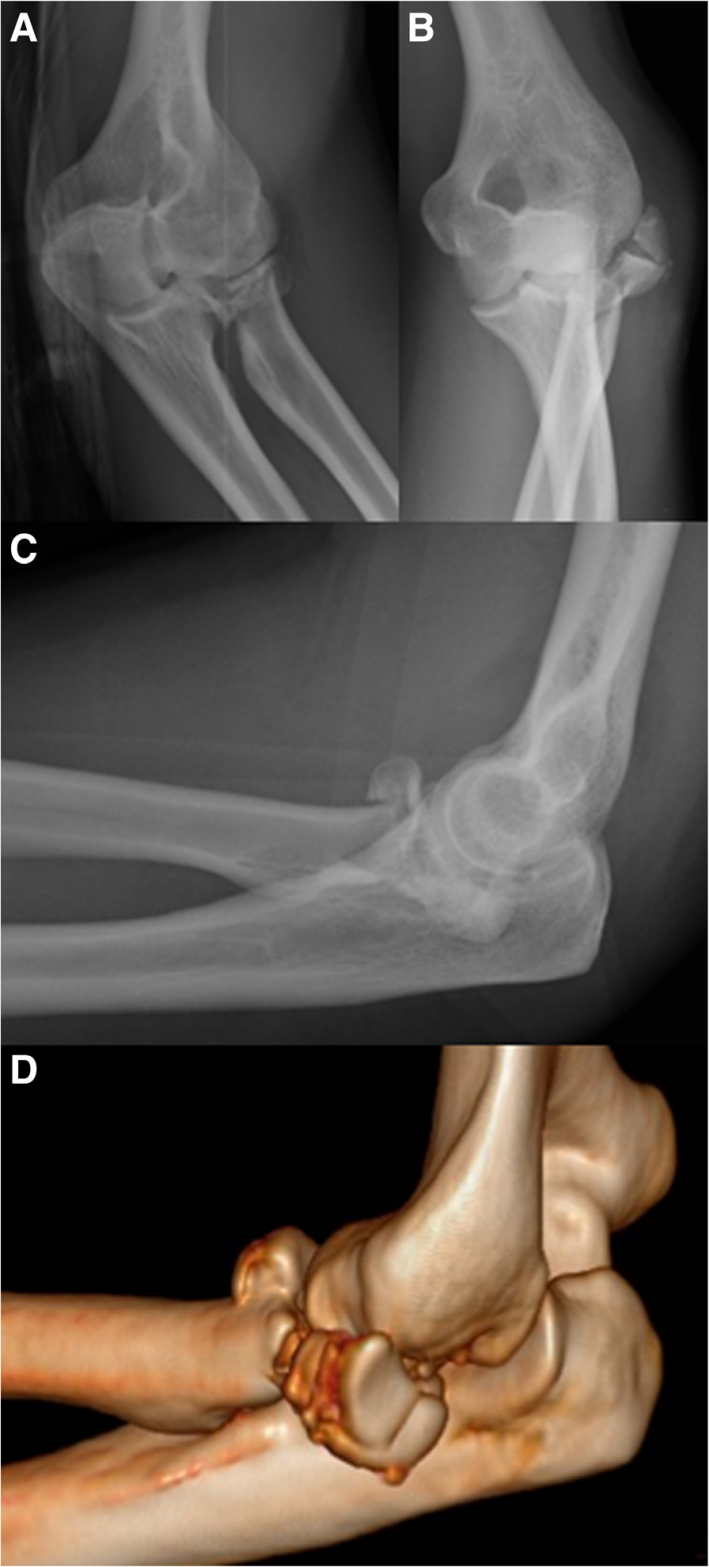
Clinical case 4, Acute Engaged ELI, pre-operative: elbow. Pre-operative left elbow X-rays (a, b, c) and 3D reconstruction CT scan (d) images showing a Mason 3 radial head fracture
